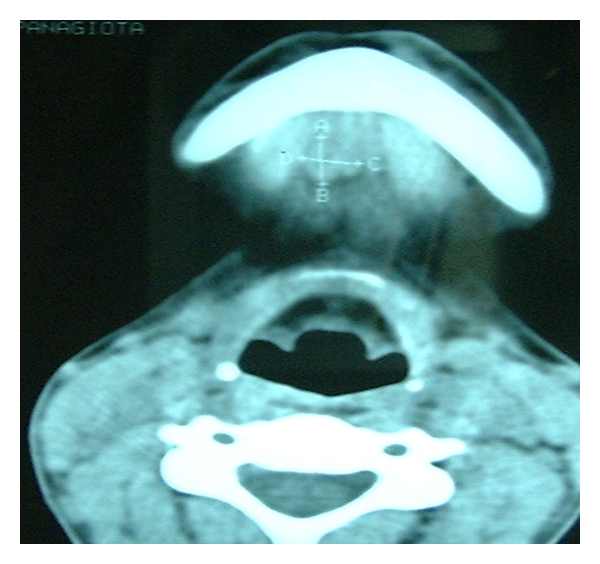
The computed tomography (CT) of the submental area showing a semitransparent cystic formation with distinct boundaries.
